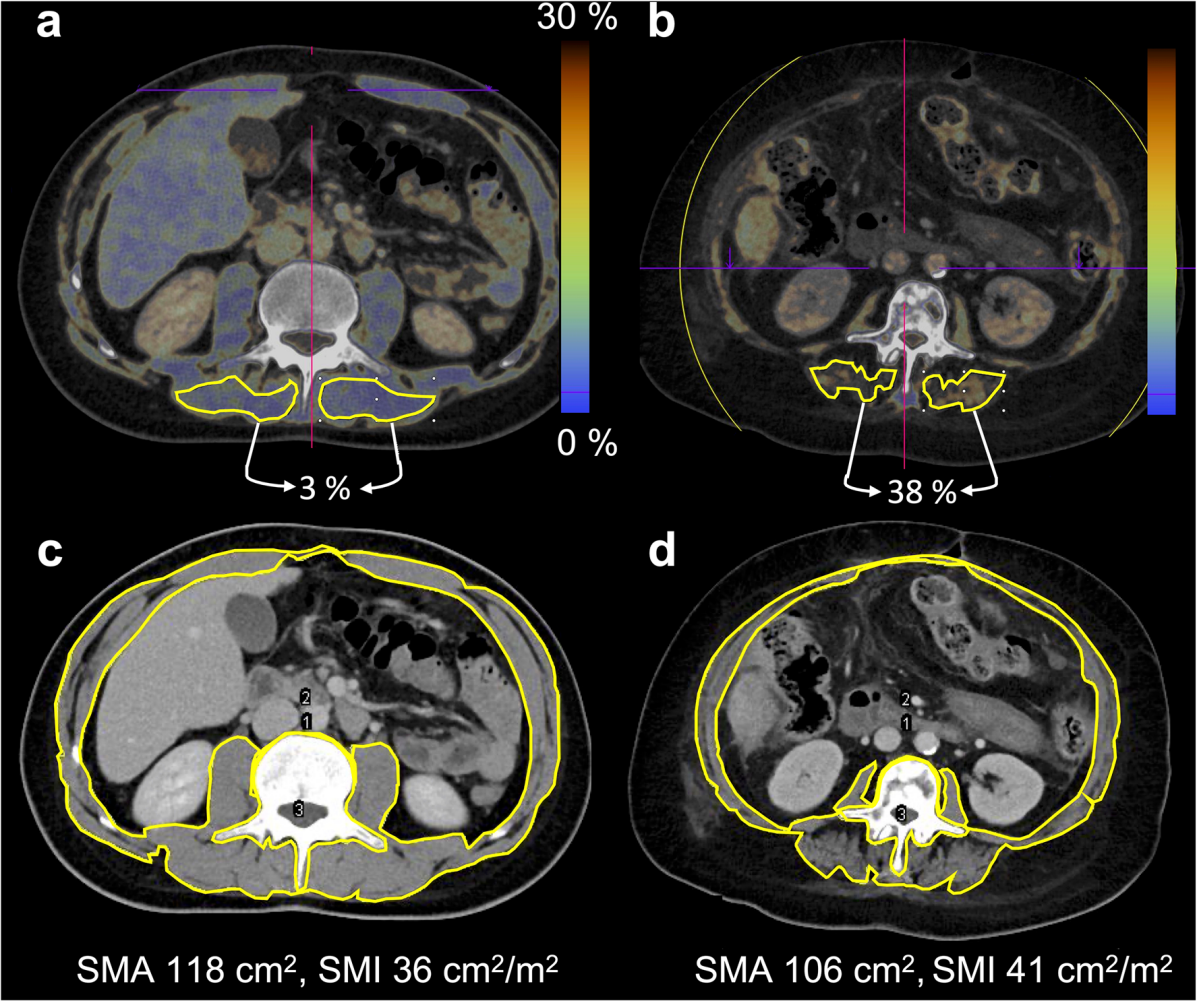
Determination of DECT fat fraction, DECT VNC HU, and SMA. Dual-energy computed tomography fat fraction (DECT-FF) and DECT virtual non-contrast-enhanced Hounsfield units (DECT VNC HU) were acquired from regions of interest (ROIs) of the posterior paraspinal muscles (m. erector spinae) (a, b). The skeletal muscle area (SMA) was determined after delimitation of muscle-specific tissue (−29 to +150 HU) by subtraction of the perimeter of the third lumbar vertebra and the inner skeletal muscle perimeter from the outer skeletal muscle perimeter [18] (c, d). The skeletal muscle index (SMI) was derived by SMA/height^2^. Two patients with low muscle fat percentage of 3% in DECT-FF and low SMI (a, c: 32 y, m, no pre-existing conditions) and high muscle fat of 38% in DECT-FF and higher SMI (b, d: 68 y, f, metastasized breast cancer) are exemplarily shown
